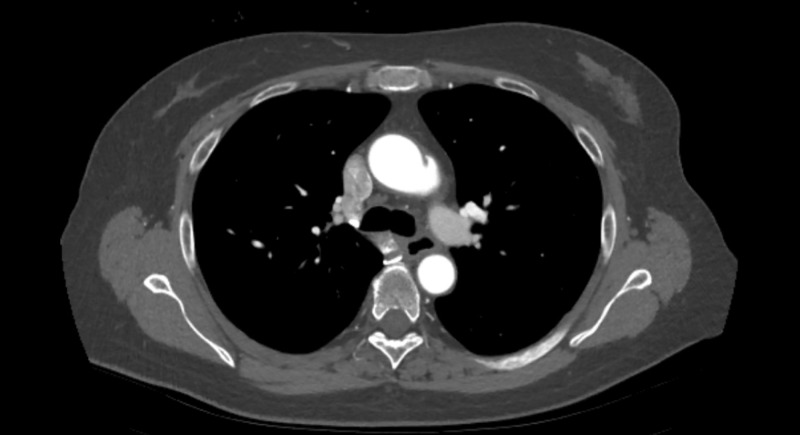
Follow-up CT chest Follow-up computed tomography (CT) chest demonstrating the attenuation of inflammation. The pseudoaneurysms were believed to be sequelae of the initial episode. They have been stable on further repeat imaging.
